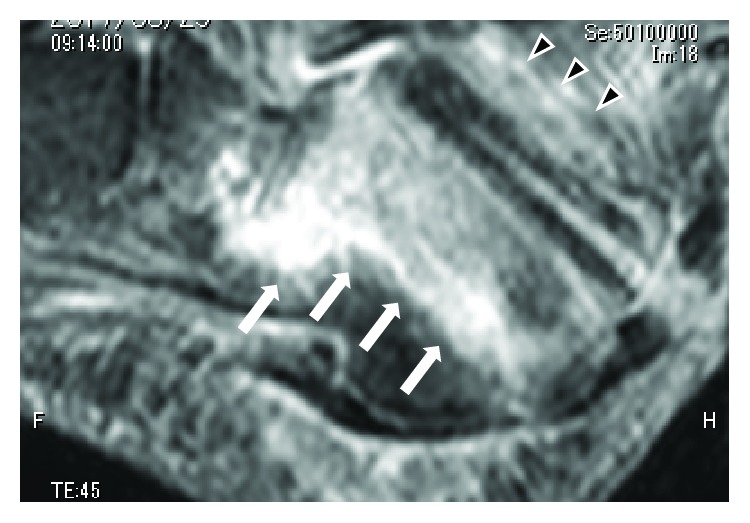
Fat suppressed T2-weighted left ankle magnetic resonance sagittal images before the 2nd operation. High-intensity area was confirmed around the pin tract (black triangle) and body of calcaneus (white arrow). Abscess formation was suspected.
